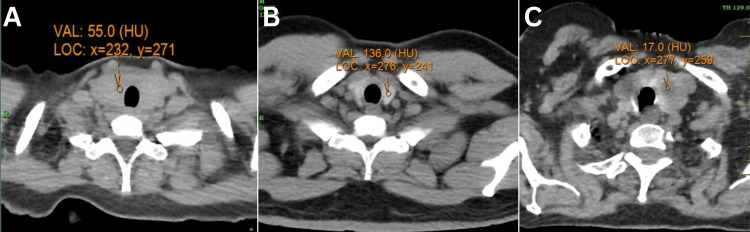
CT thyroid images. (A) Enlarged thyroid gland with diffuse hypodensity, 55 HU (<70); (B) normal thyroid gland, 136 HU (>70); (C) multiple hypodense nodules are seen in both lobes of the thyroid gland. The left lobe is enlarged due to the presence of the nodules.
